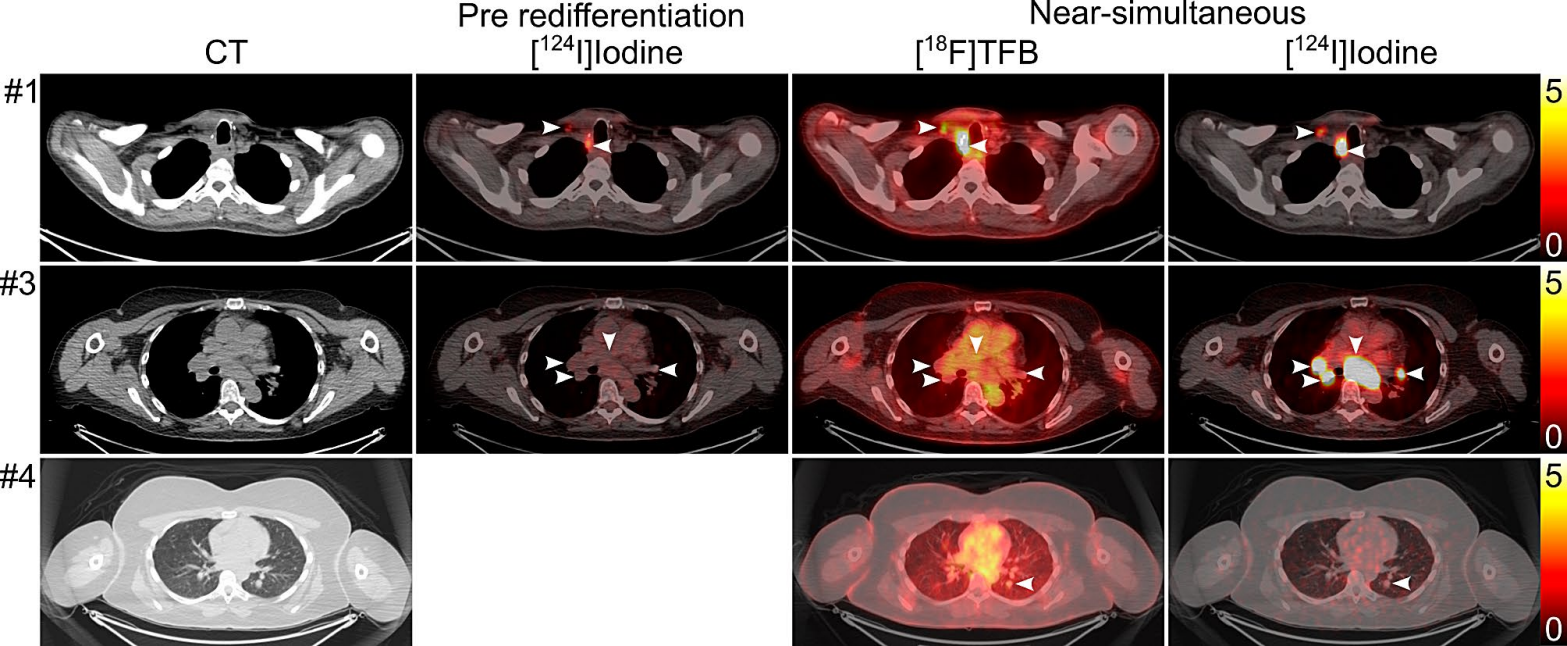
First and second row: transversal CT (first column), corresponding pre-redifferentiation [^124^I]Iodine PET/CT fusion (if available, second column) and after near-simultaneous tracer application, [^18^F]TFB PET/CT fusion (third column), and [^124^I]Iodine PET/CT fusion (fourth column). First row, patient 1, example of concordant lesions: Right paratracheal local relapse and right neck nodal metastasis (arrowheads) demonstrate increased [^124^I]Iodine uptake after redifferentiation therapy. Both lesions were well visible in [^18^F]TFB. Second row, patient 3, example of better lesion detection with [^124^I]Iodine: At baseline non-[^124^I]Iodine-avid mediastinal, hilar, and lung metastases demonstrated intense uptake after redifferentiation therapy with SUV_max_ up to 285.0 (arrowheads). Lesions did not demonstrate tracer uptake above blood pool in near-simultaneous post-redifferentiation [^18^F]TFB PET/CT. Third row, patient 4, example of better lesion detection with [^18^F]TFB: A left lower lobe lung metastasis well visible in CT did not demonstrate [^124^I]Iodine uptake above background noise, but featured mild focal [^18^F]TFB uptake. All images are displayed as SUV 0–5
